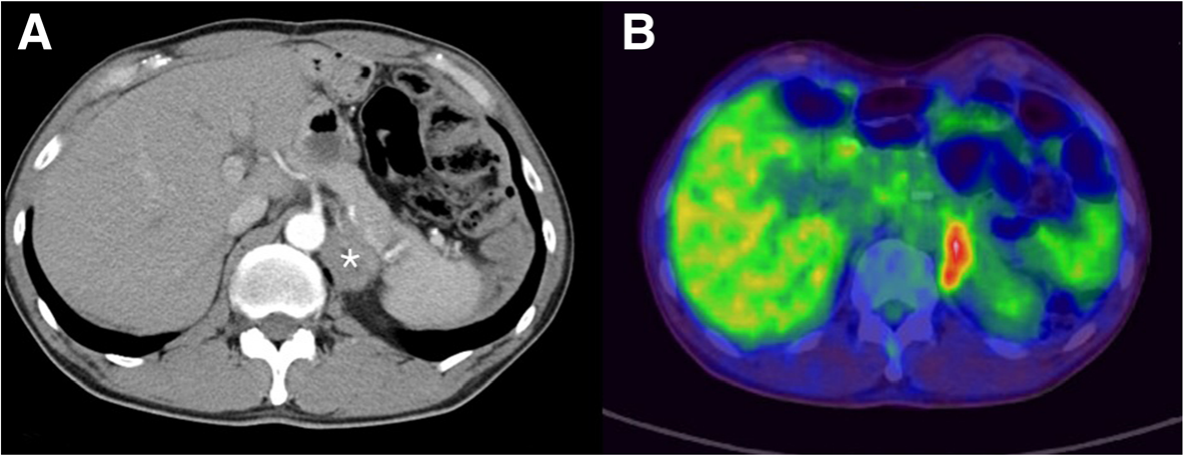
Follow-up computed tomography and positron emission tomography–computed tomography scans of patient 1 obtained in September 2011 show a 3-cm left adrenal mass (asterisk). (A) Computed tomography shows accidentally detected left adreanal mass. (B) PET-CT shows significant FDG uptake area correlated with enlarged left adenal mass.
